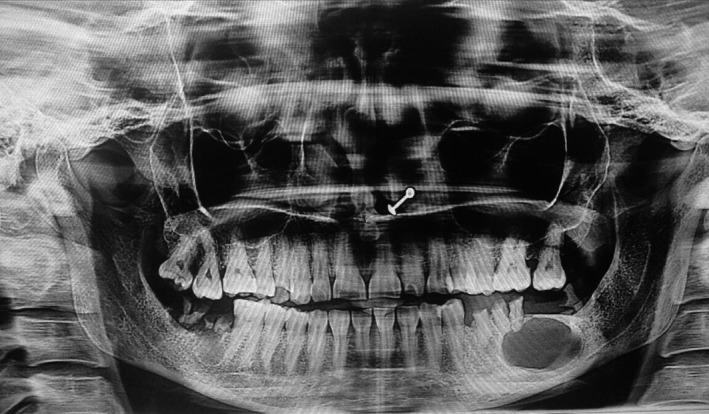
Orthopantomogram showing unilocular radiolucency involving root stumps of left mandibular first molar
